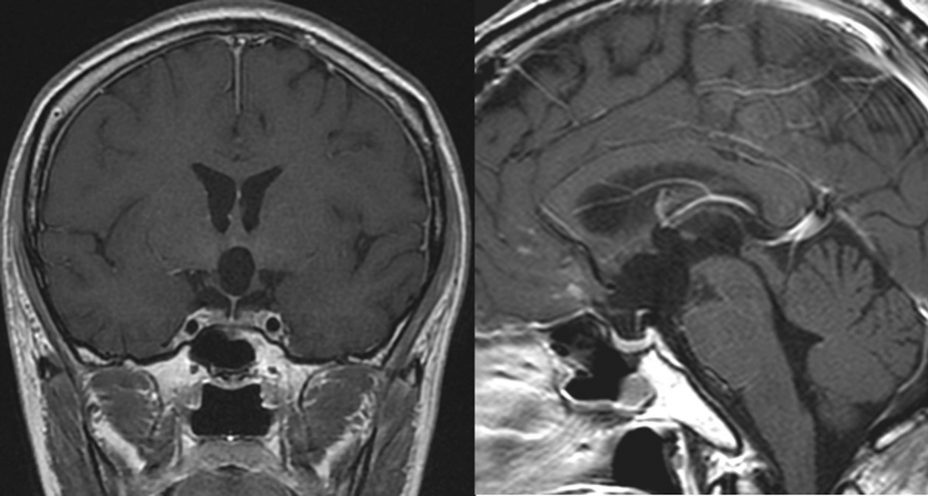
Post-radiotherapy MRI demonstrating a complete remission of the germinoma lesions.
